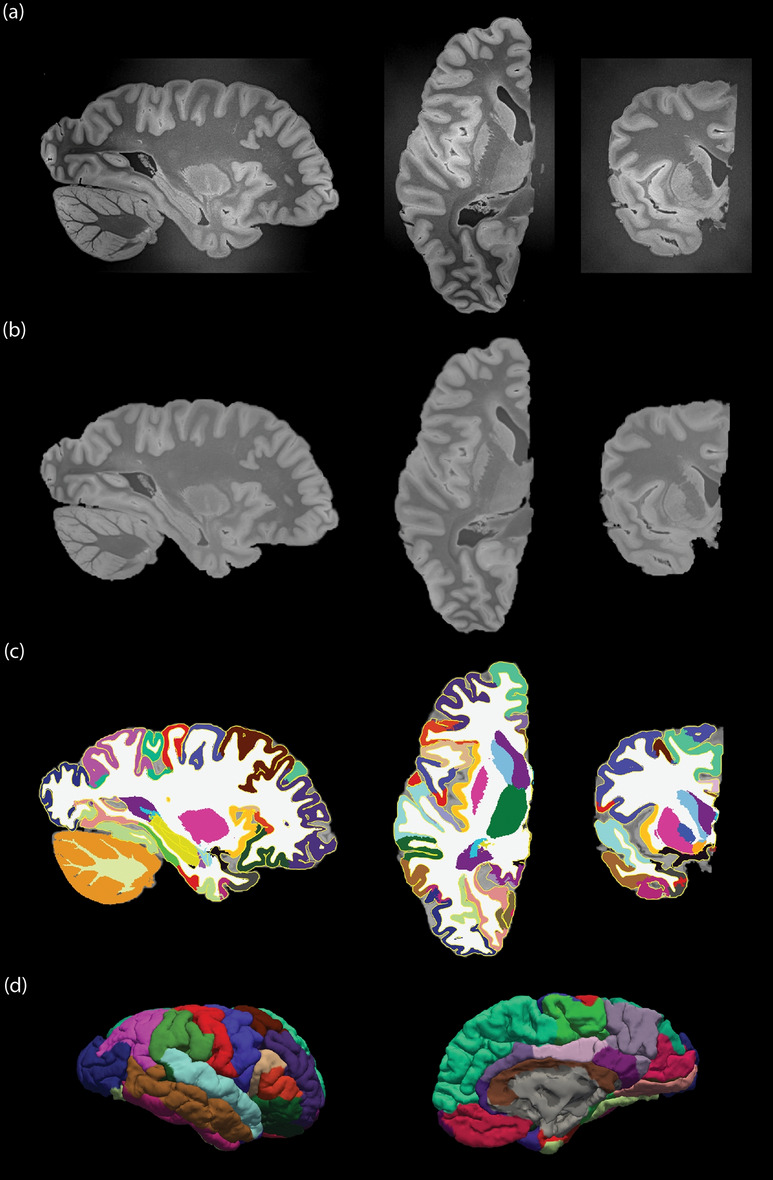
Overview of the main MRI preprocessing steps. (a) The raw ex vivo MRI data as acquired, showed in sagittal (left), axial (middle) and coronal (right) views; (b) brain extraction and bias field correction given by SAMSEG; (c) segmentation or subcortical structures given by SAMSEG, combined with cortical segmentation and parcellation provided by FreeSurfer, with the outline of the reconstructed surfaces in yellow; (d) 3D rendering of the cortical surface from lateral (left) and medical (right) views. The color coding of the segmentation and parcellation follows the FreeSurfer convention.
